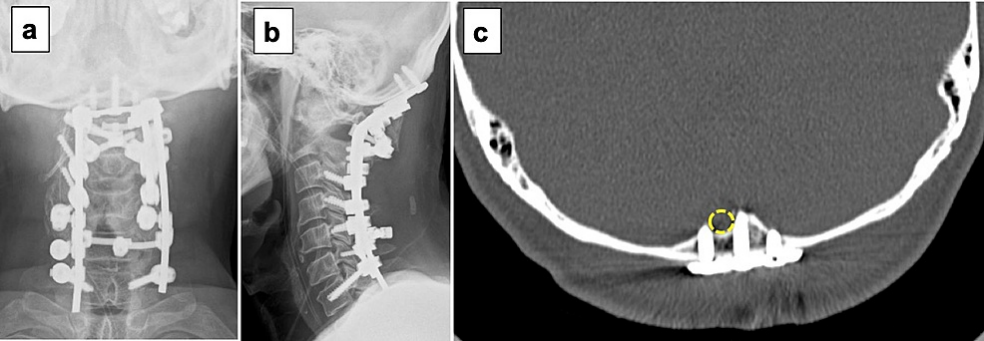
Postoperative images (a, b) Postoperative radiographs showing posterior occipito-C7 fusion. (c) Postoperative axial CT showing bi-cortical occipital screw placement avoiding the prominent occipital venous sinus (dotted circle) and no intracranial hematoma.
